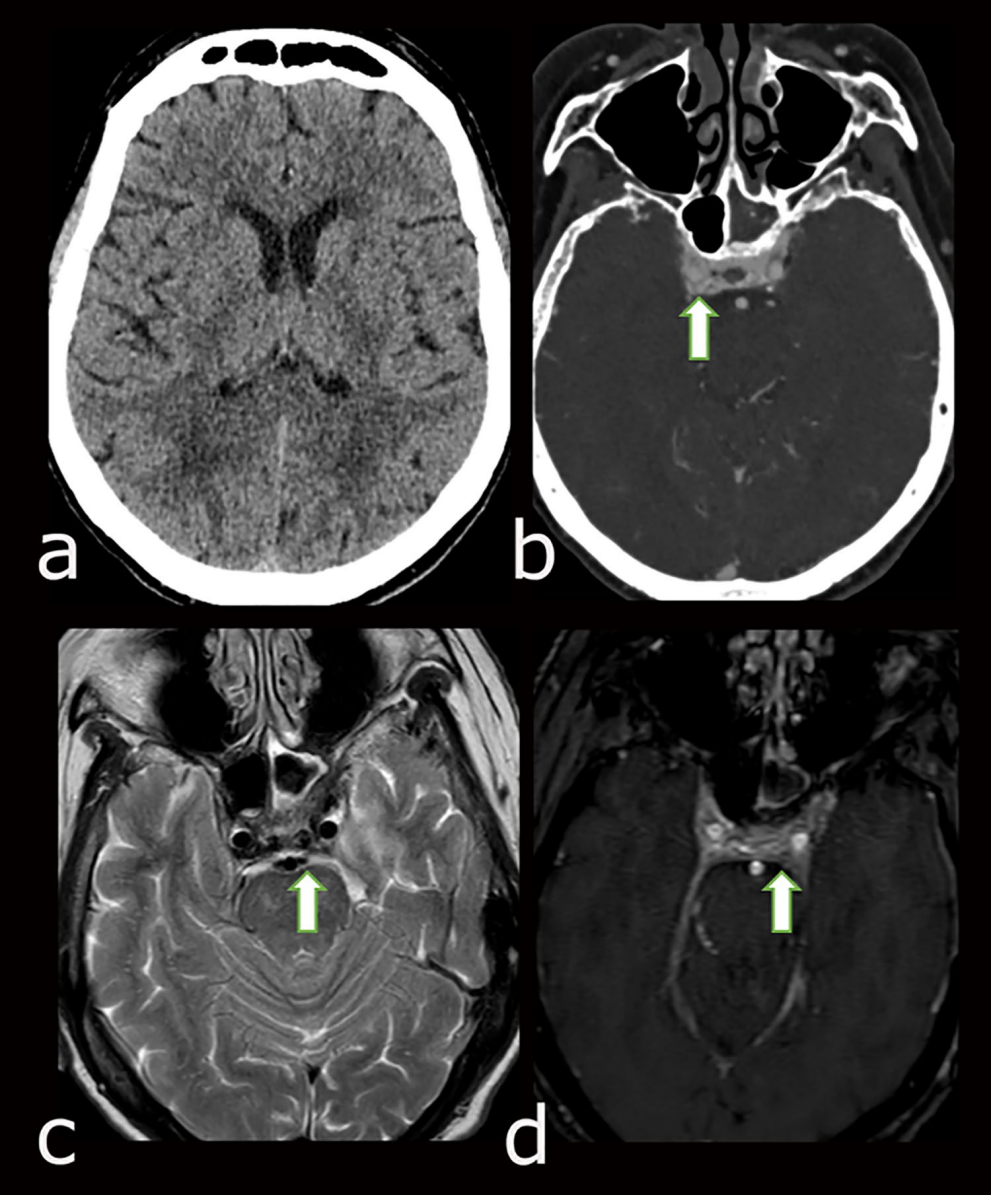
Cross-sectional imaging workup. (a) Initial non-contrast head CT excluded
